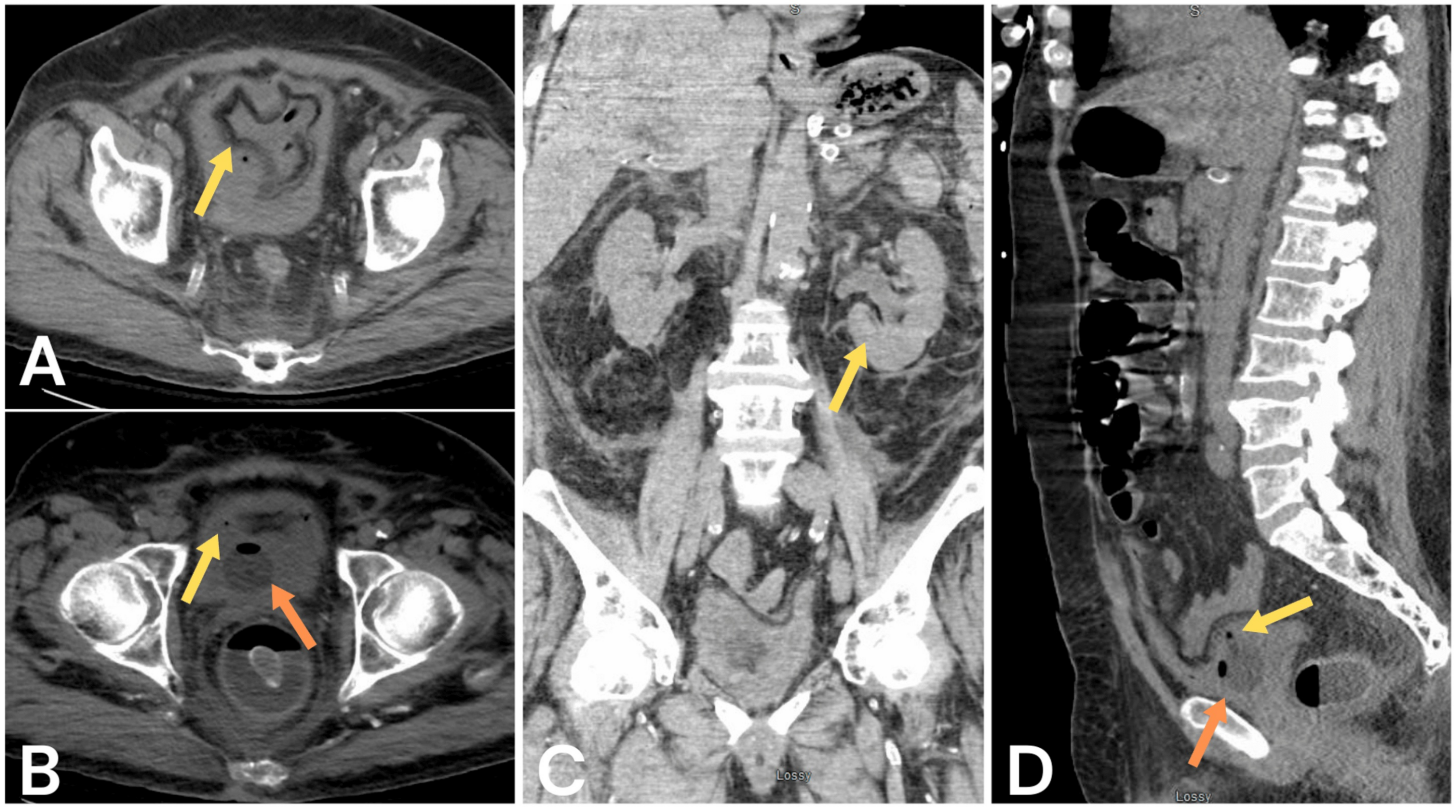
CT imaging without contrast demonstrating (A) axial view of irregularly shaped bladder with evidence of air in the wall, (B) axial view of air in the bladder wall (yellow) and with a Foley balloon in place (orange), (C) coronal view of hydroureteronephrosis of the left kidney, and (D) sagittal view of irregularly shaped bladder with air in the wall (yellow) and Foley balloon in place (orange).
